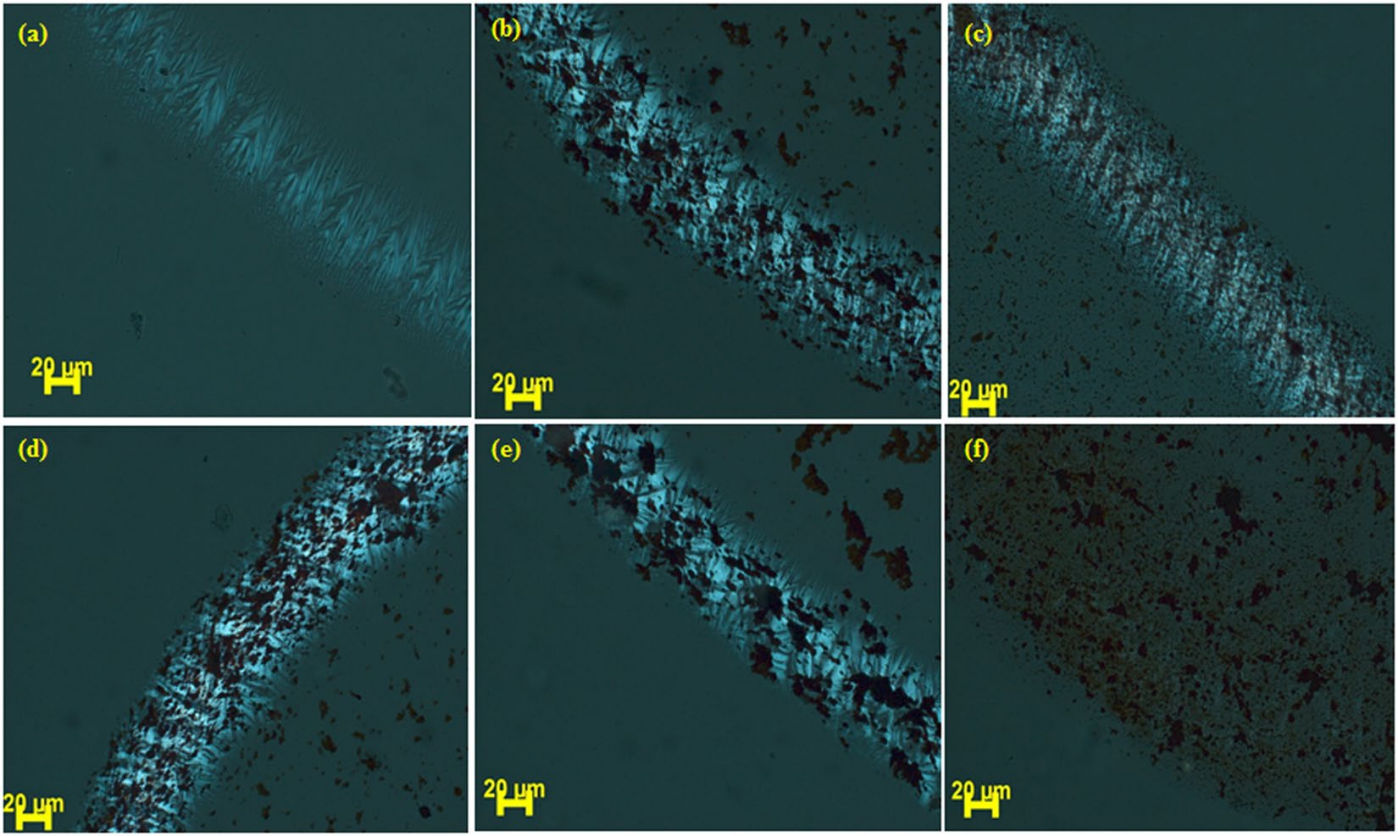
POM images. (a) Top view of CT-DNA only, (b–f) CT-DNA with hematite NPs 2.5 mg/ml, 5 mg/ml, 10 mg/ml, 20 mg/ml, 50 mg/ml respectively.
